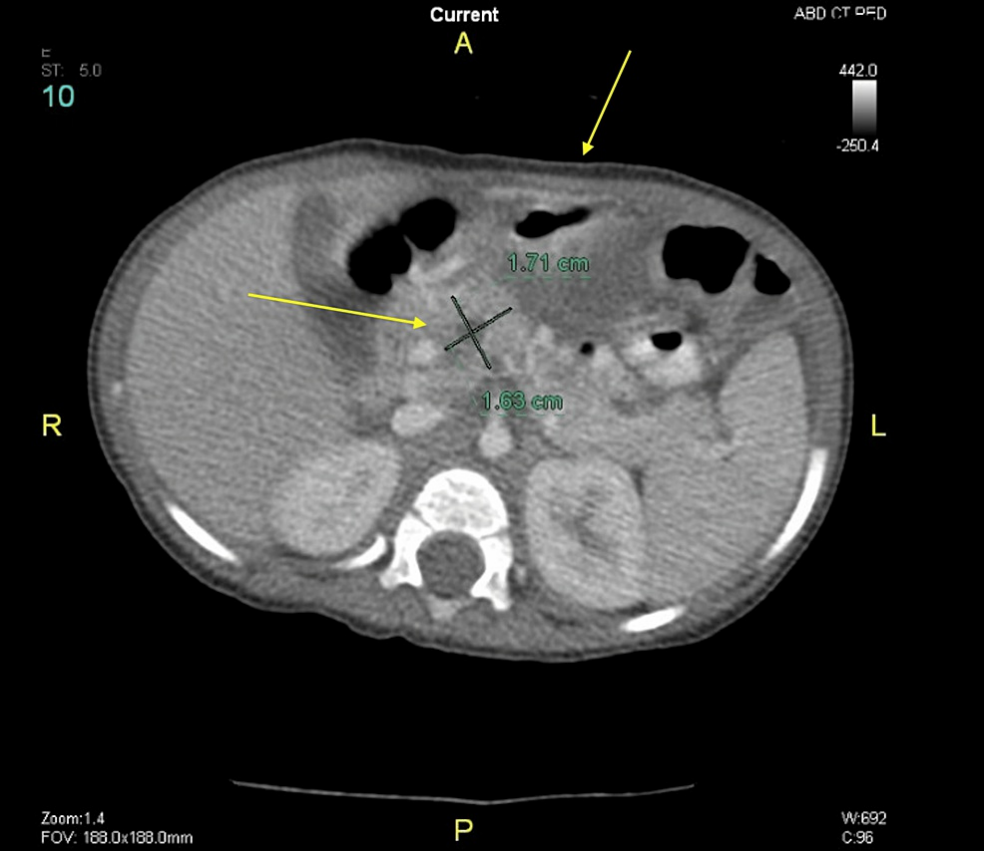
Abdominal CT scan showing pseudocyst in the head of the pancreas (arrows) CT: computed tomography
